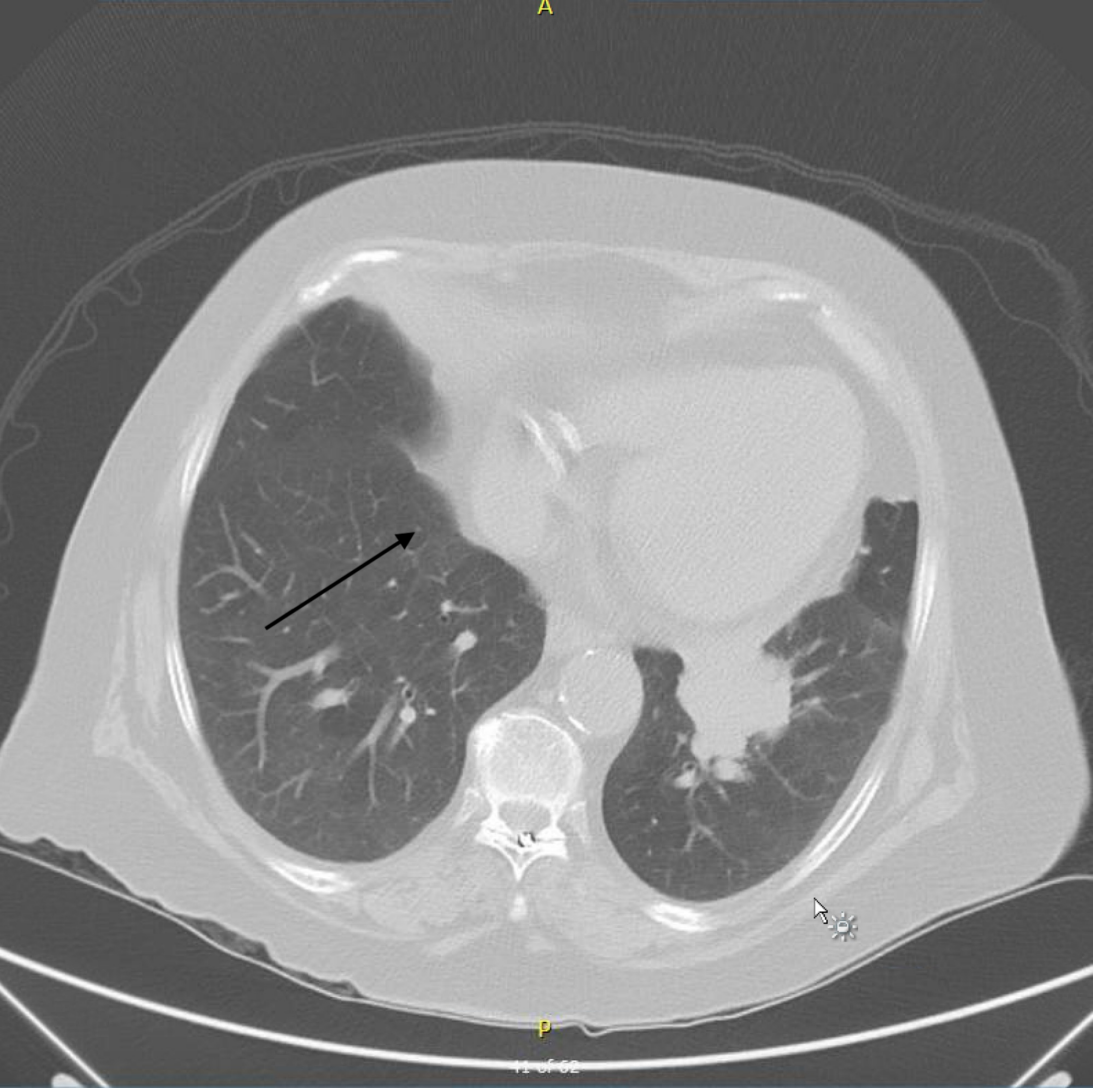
Chest CT showing mass-like consolidation surrounded by nodules.
